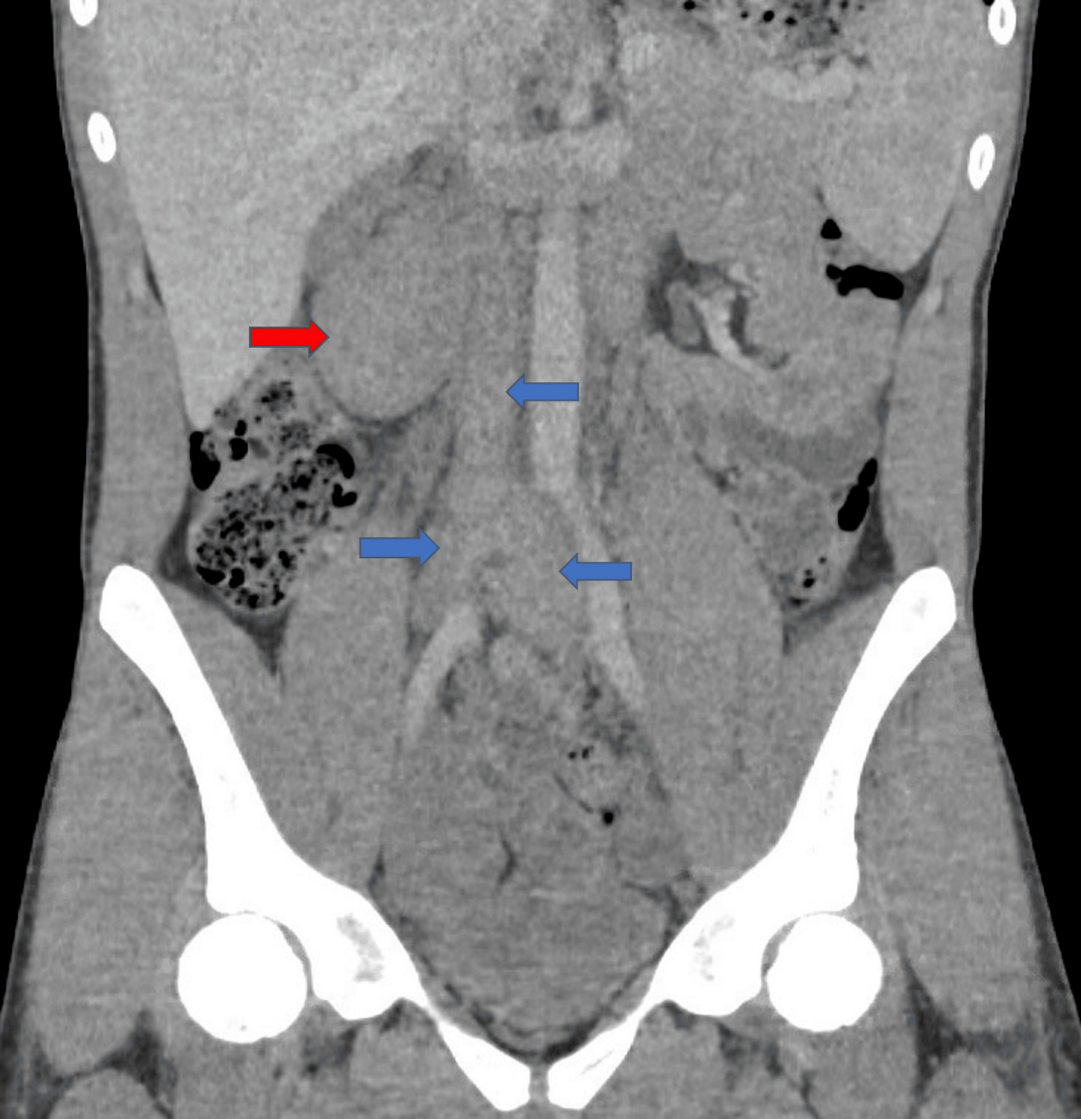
CT of the abdomen and pelvis demonstrating a large retroperitoneal mass (red arrow) and venous thrombus throughout the infrarenal inferior vena cava, and common right and left iliac veins (blue arrows).
